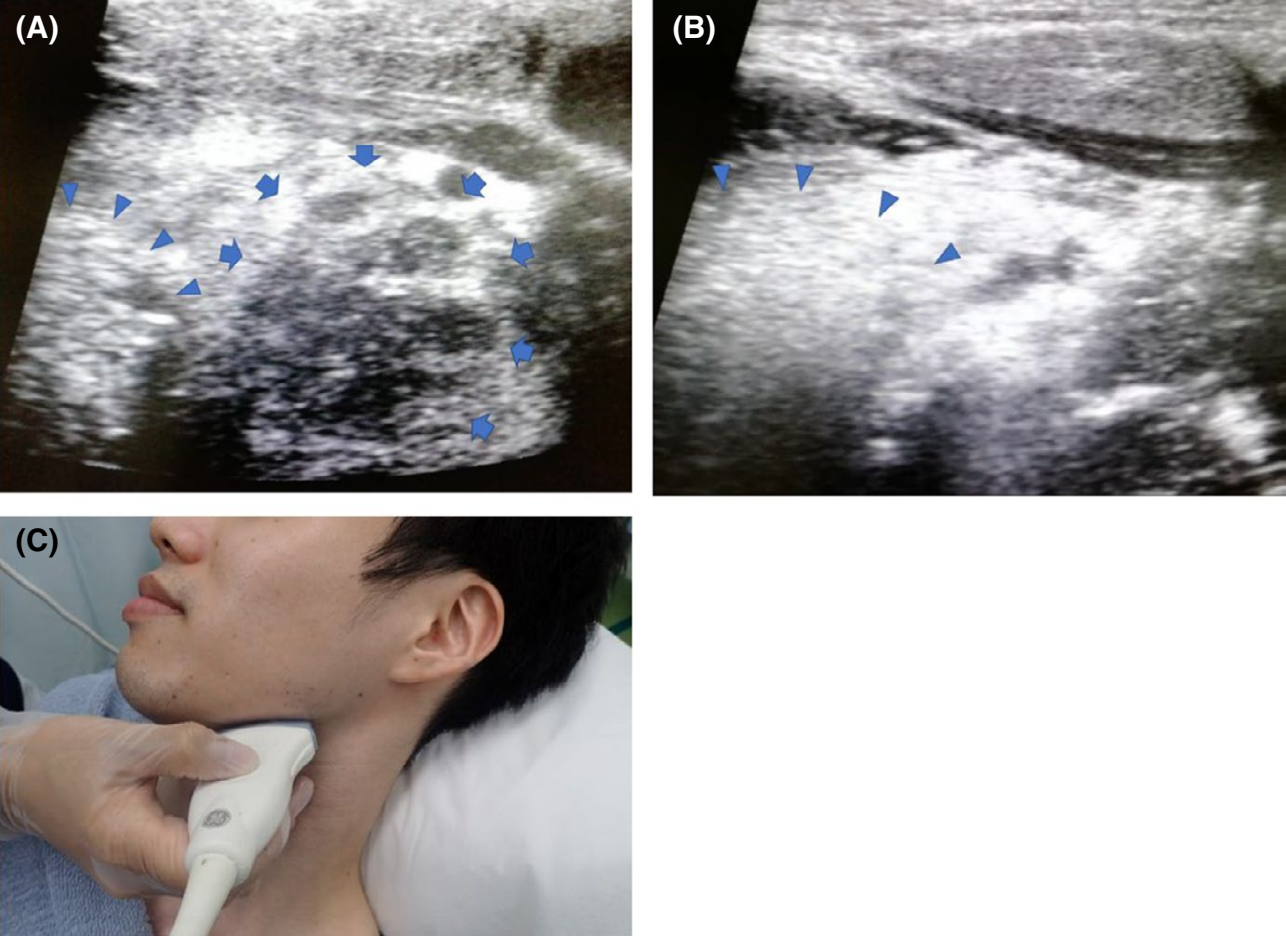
The patient was asked to move their tongue (arrowhead) to improve imaging of the tonsil. A swollen palatine tonsil and low‐intensity abscess measuring 25 mm in size (arrow) can be seen behind the tongue (A, Video [Link], [Link]). Ultrasonography image of the unaffected side; the right palatine tonsil is not swollen (B, Video [Link], [Link])
